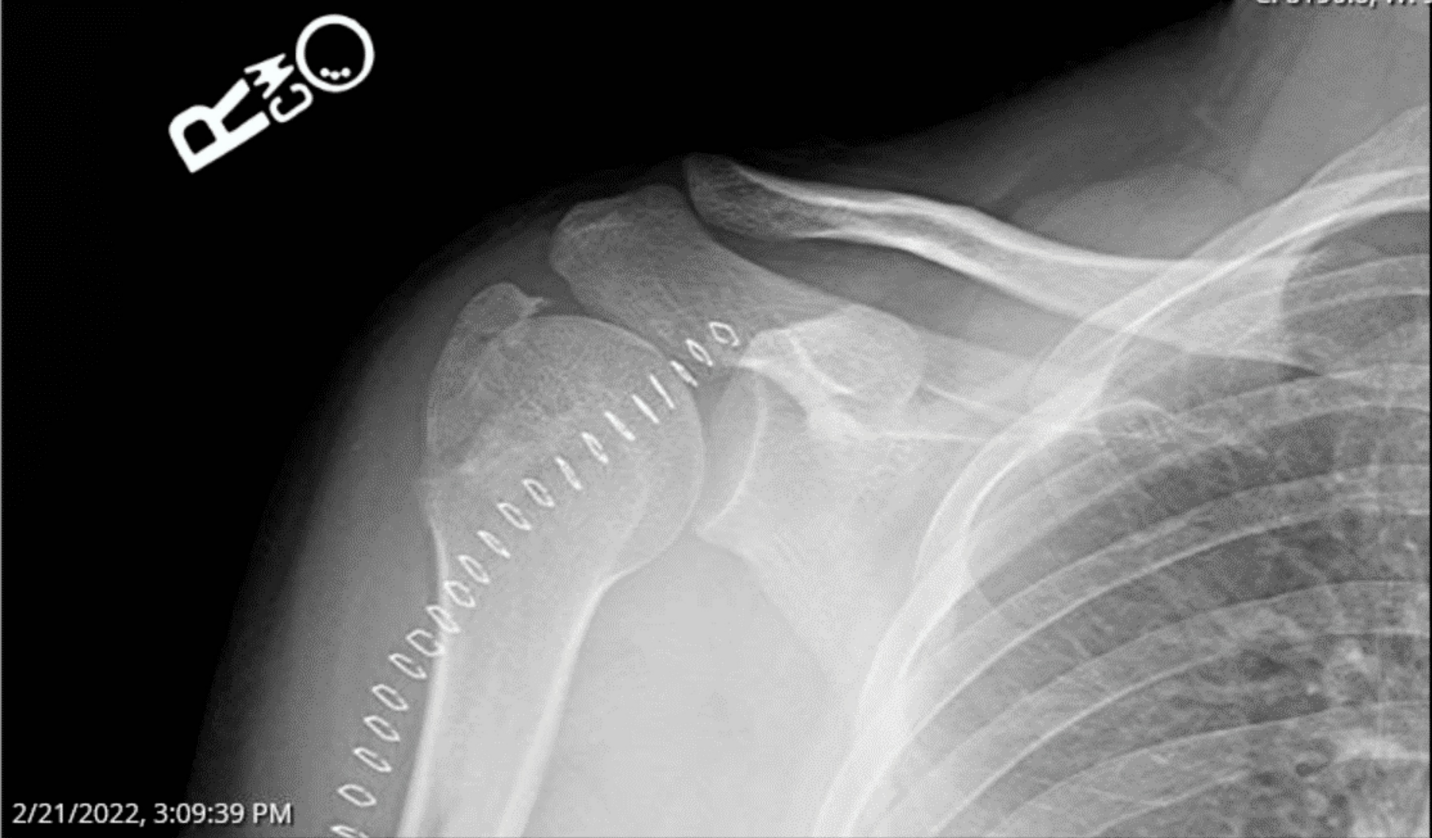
X-ray showing greater tuberosity fracture following open reduction and internal fixation
